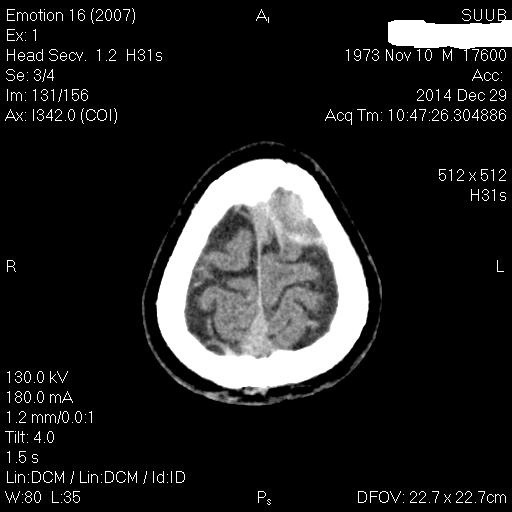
Axial CT scan which evidences a left frontal serohematic effusion in patient M.F. of 41 years old
